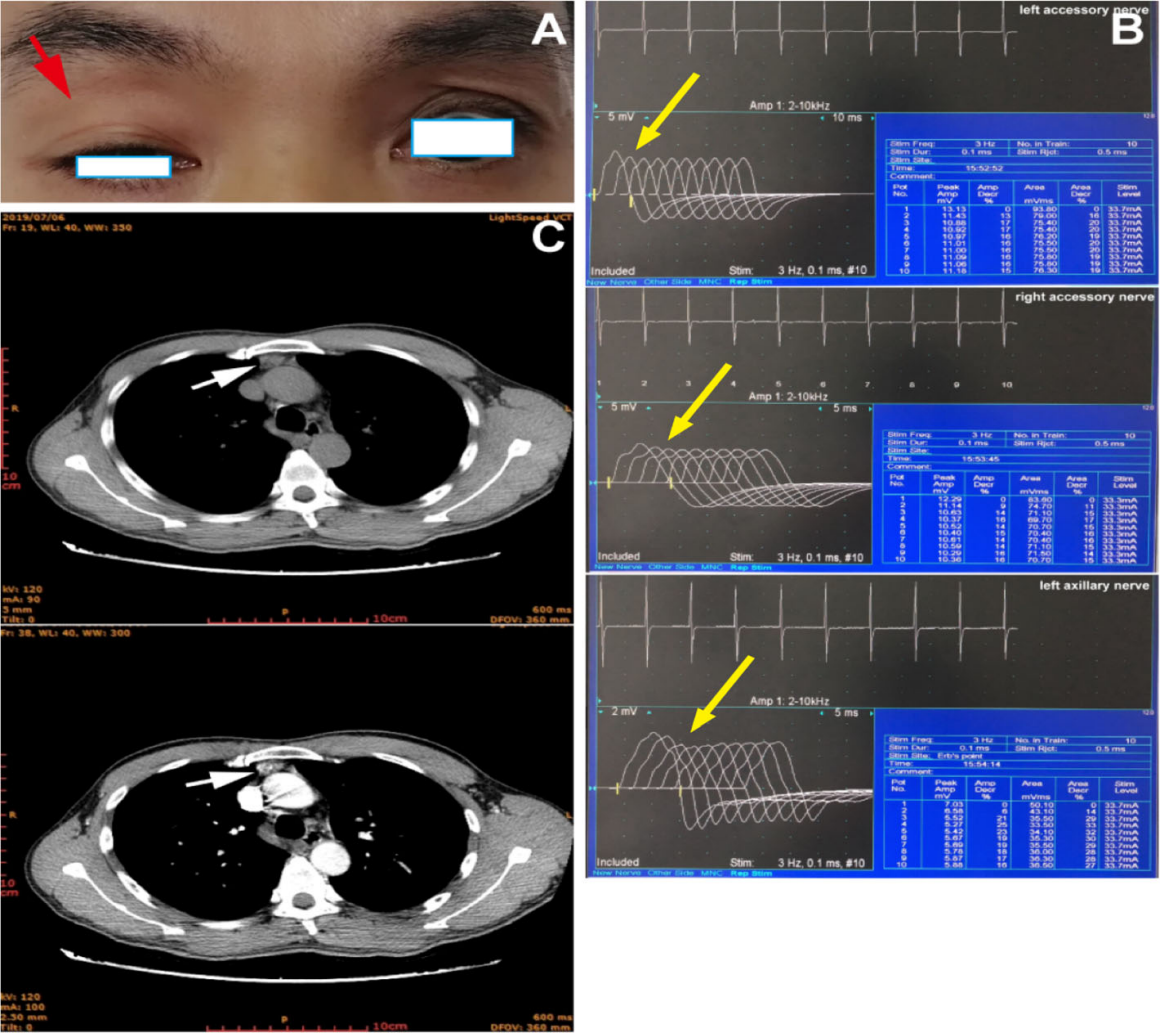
Manifestations and auxiliary examinations of myasthenia gravis. a Ptosis of the right upper eyelid (red arrow); b repetitive nerve stimulation test results of the bilateral accessory nerve and left axillary nerve were positive (yellow arrows); c computed tomography images showed thymic hyperplasia (white arrows)
